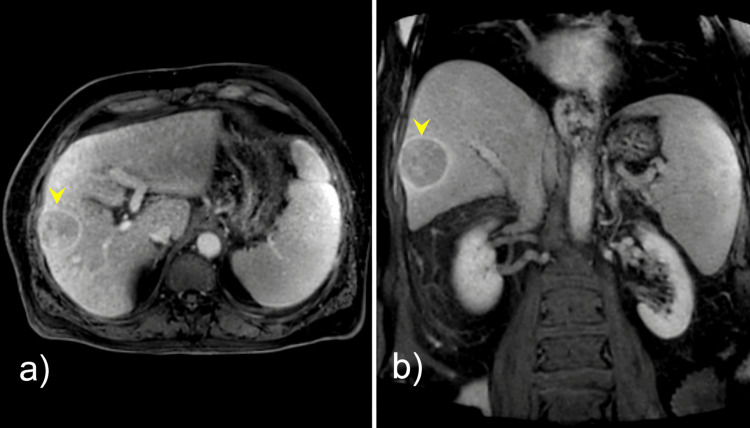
Contrast-enhanced computed tomography (CT) images of the abdomen (A) Axial and (B) coronal contrast-enhanced computed tomography (CT) images of the abdomen demonstrating cirrhotic liver morphology with a lesion (yellow arrowheads) in hepatic segment V with arterial phase hyperenhancement and washout on delayed phases, consistent with a Liver Imaging Reporting and Data System (LI-RADS) 5 lesion.
